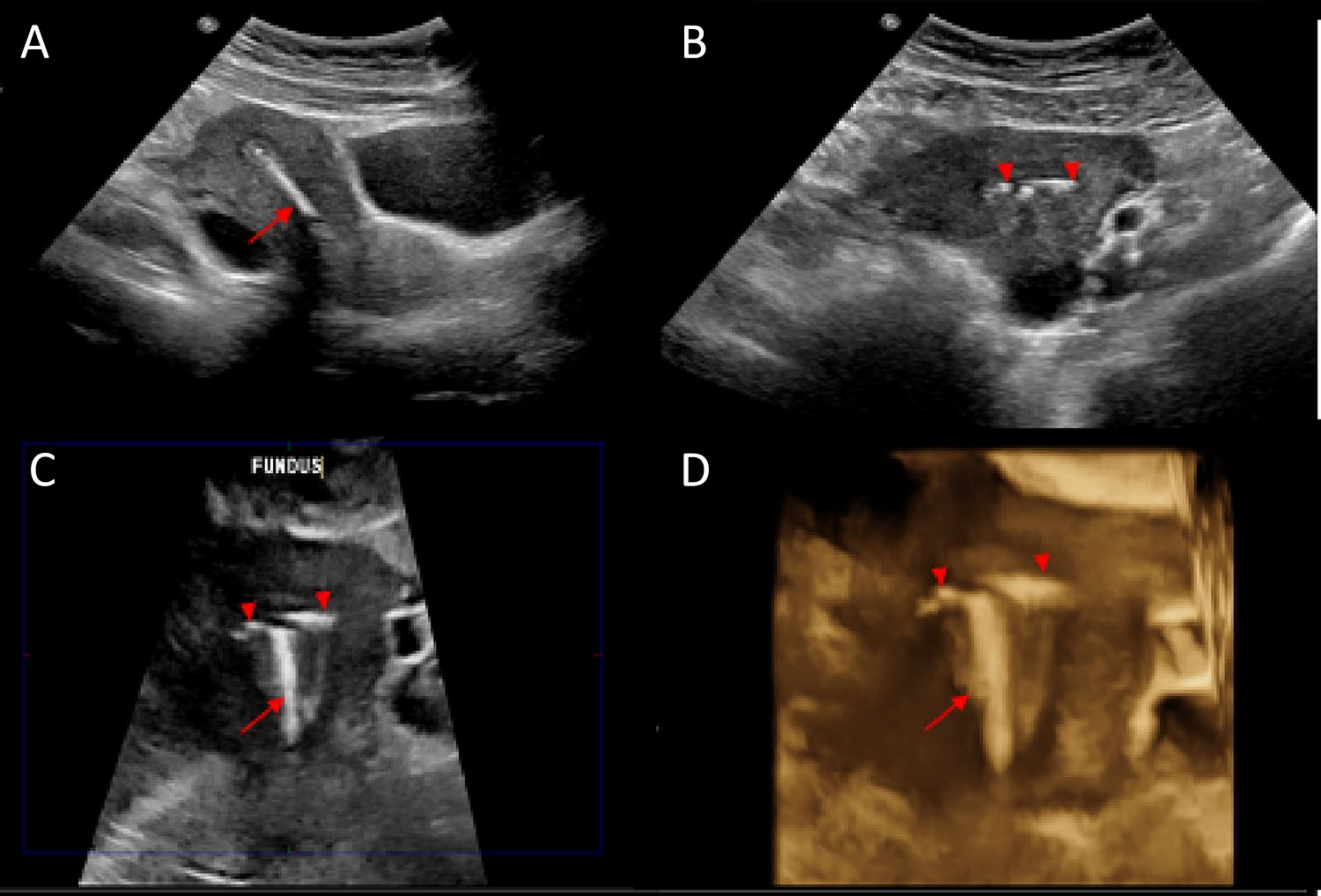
Proper IUD placement. 2D grayscale transabdominal ultrasound images of the uterus in long (A) and short axis (B) demonstrating an IUD stem (arrow) centered within the endometrial cavity and both arms (arrowheads) of the device extending laterally at the uterine fundus. 3D grayscale (C) and gynecologic tissue rendered (D) ultrasound images of the same patient confirming appropriate positioning of the stem and arms of the IUD
